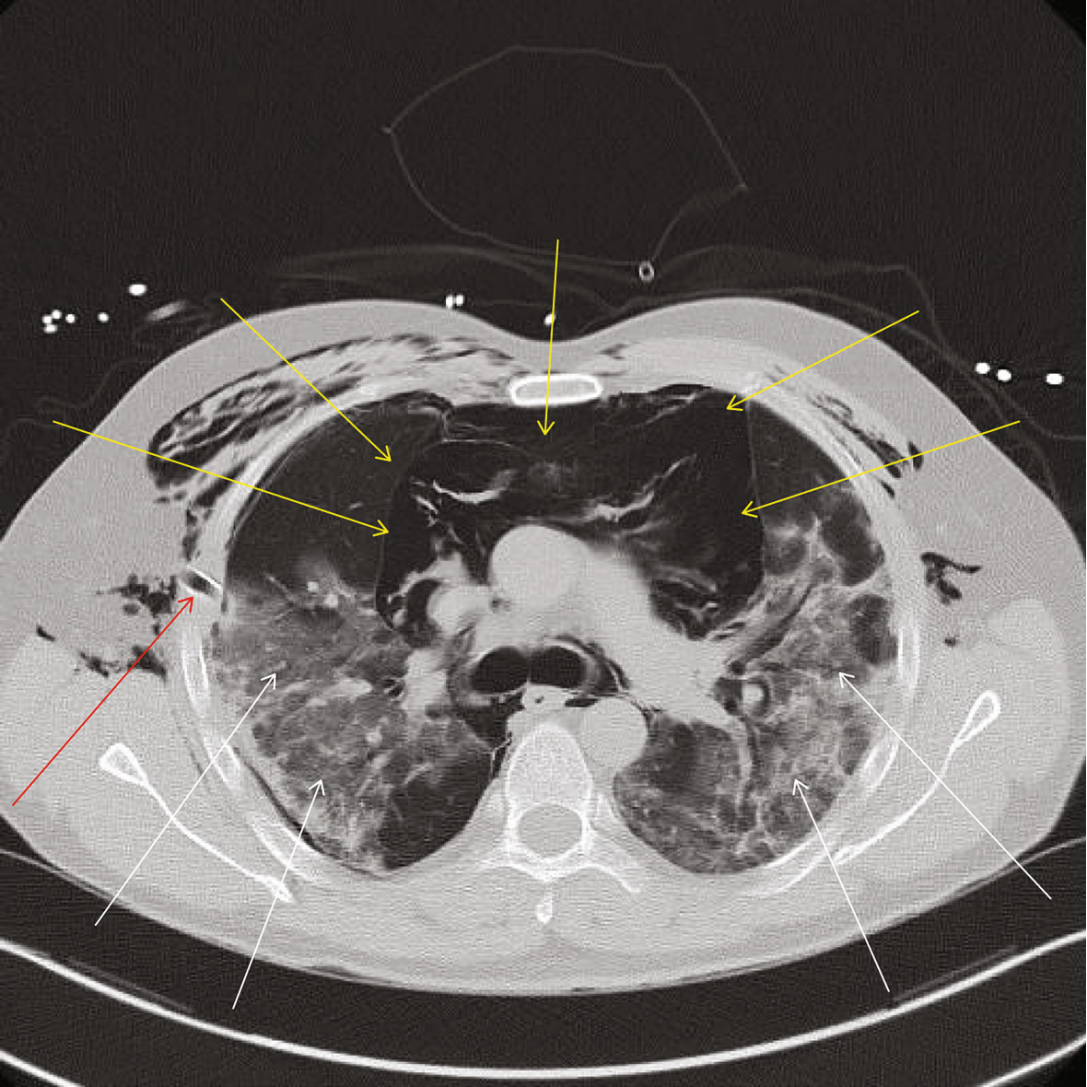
Initial chest CT-scan at the level of tracheal bifurcation directly after transfer to our ICU. The white arrows indicate bilateral ground glass opacities. Yellow arrows display the pneumomediastinum. The red arrow points at the thoracic drainage which was placed during the stay in the referring hospital.
